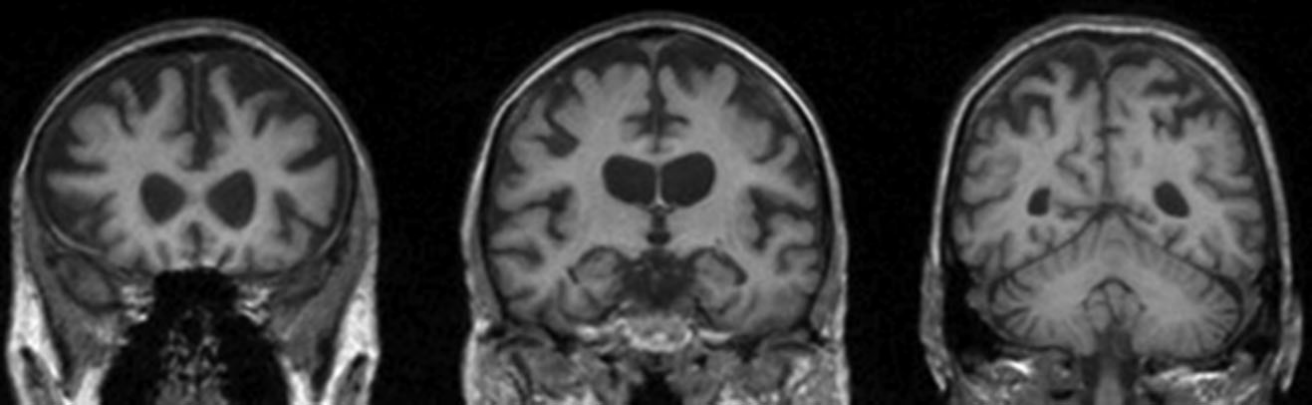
MRI scan of case 2.1. Showing symmetrical atrophy of the hemispheres and enlarged lateral ventricles.
